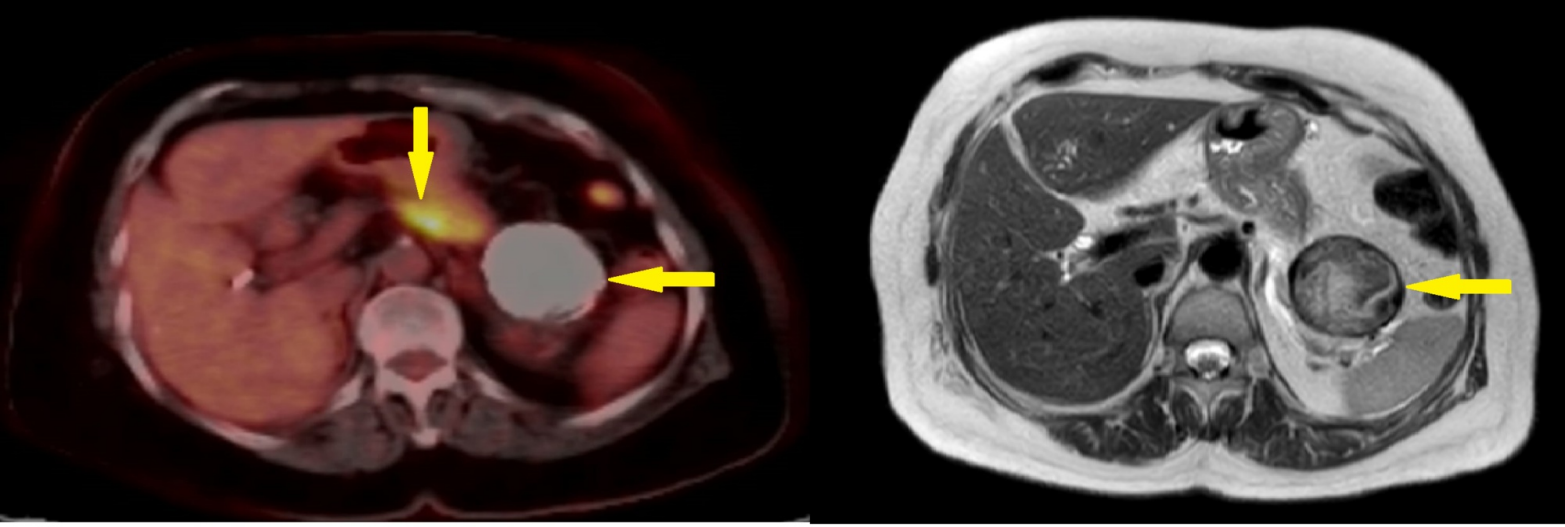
PET-CT fusion and MR images demonstrating pancreatic lesions Solid and cystic lesions in the body and tail of pancreas (arrows). PET-CT: positron emission tomography-computed tomography; MR: magnetic resonance.
